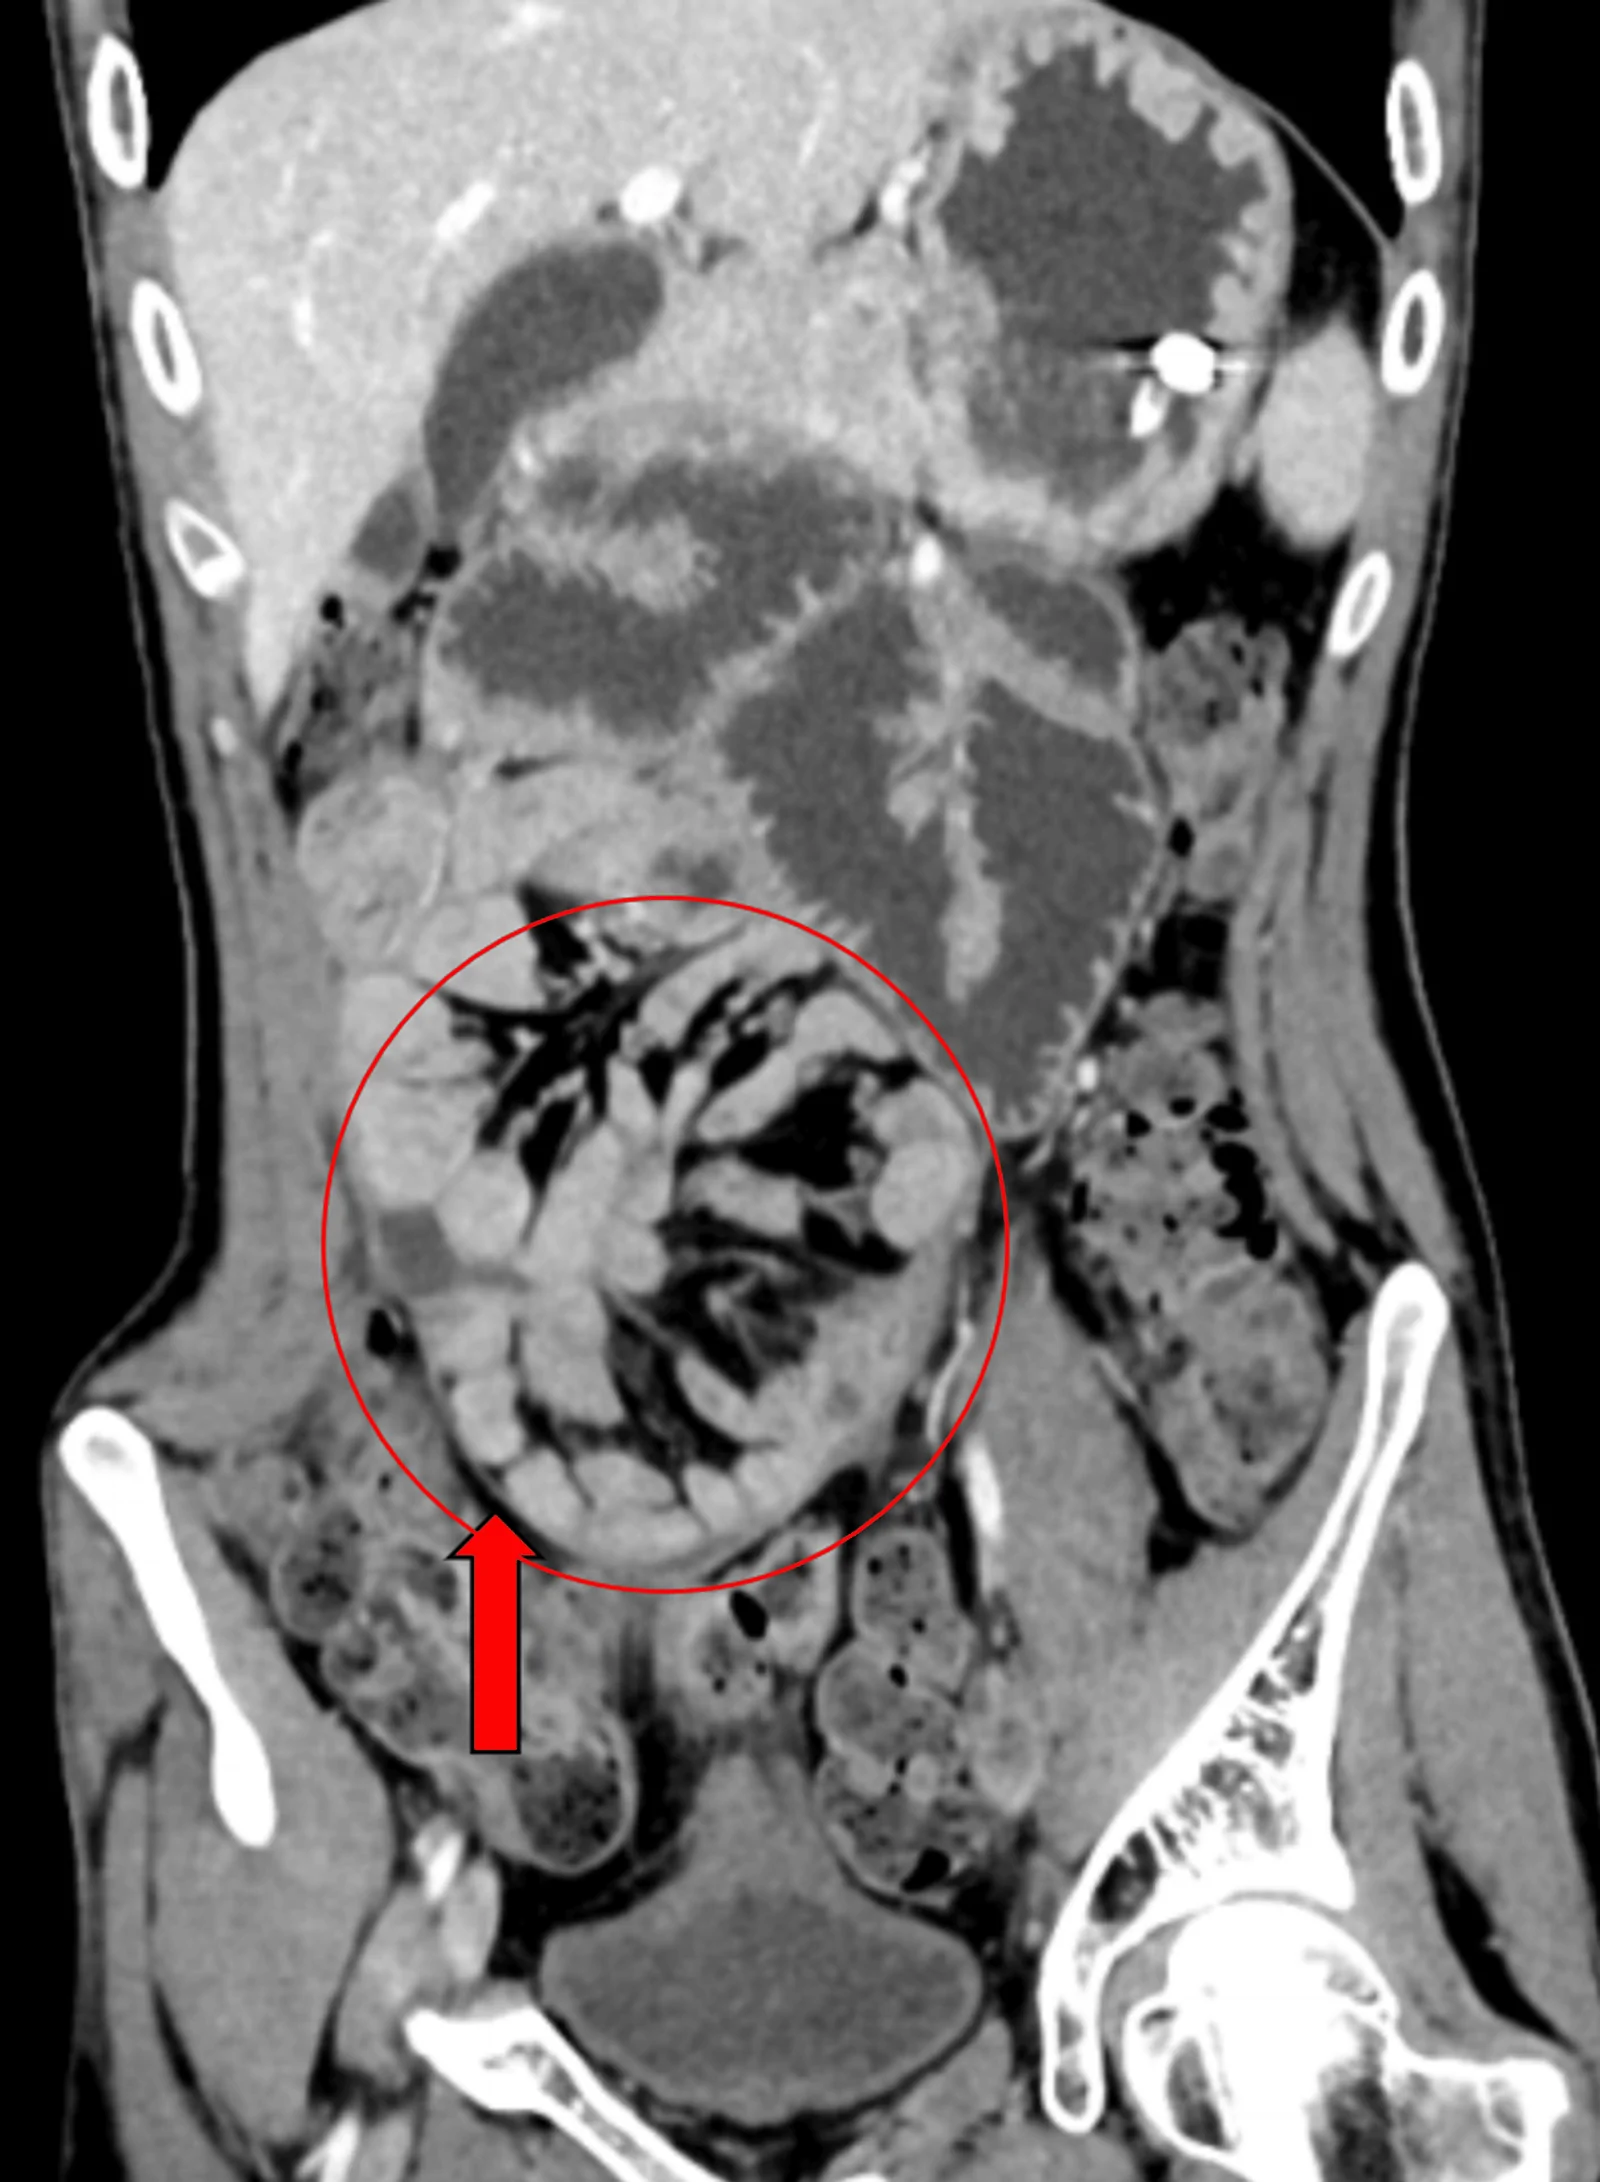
CECT features of EP (abdominal cocoon syndrome) Contrast-enhanced coronal CT image of the patient's abdomen demonstrates a cluster of mildly dilated small bowel loops (red circle) confined to the central abdomen and enclosed by a smooth, enhancing fibro-collagenous membrane (red arrow), producing the characteristic imaging appearance of EP (abdominal cocoon syndrome). CECT: contrast-enhanced computed tomography; CT: computed tomography; EP: encapsulating peritonitis
